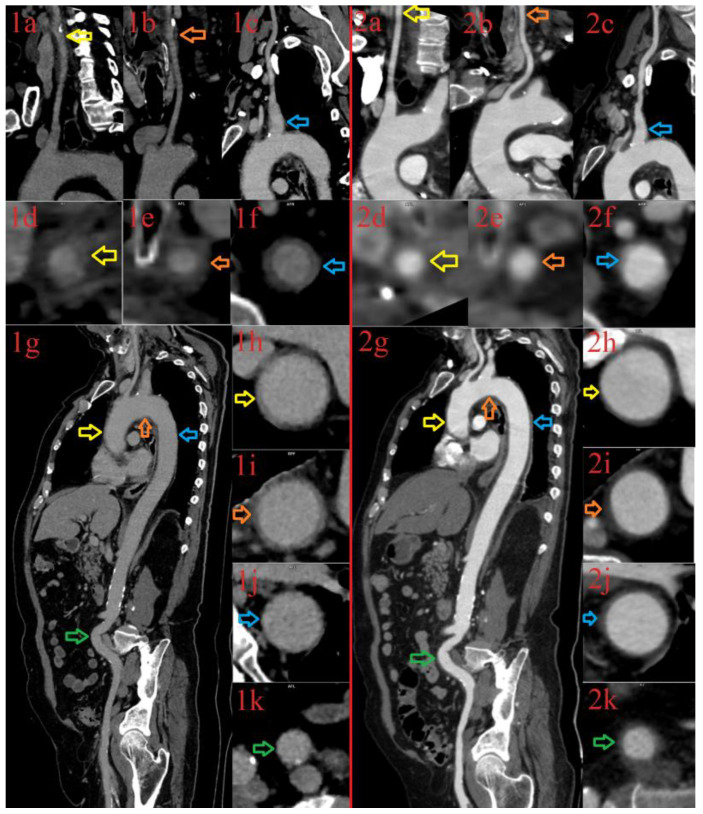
CT aortography of a 69-year-old male with large-vessel vasculitis performed at baseline (Images (1a–1k)) and at the six months follow-up examination (Images (2a–2k)). The baseline CT depicts wall thickening of the aortic arch branches that is barely seen after six months in the right carotid artery (Baseline: Image (1a,1d); Follow-up: Image (2a,2d); yellow arrows), the left carotid artery (Baseline: Image (1b,1e), Follow-up: Image (2b,2e); orange arrows), and the left subclavian artery (Baseline:: Image (1c,1f); Follow-up: Image (2c,2f); blue arrows). In addition, the baseline CT aortography depicts the inflammation of the ascending aorta (Image (1g,1h), yellow arrows) and the aortic arch (Image (1g,1i), orange arrows), which was significantly thinner after six months (The ascending aorta: Image (2g,2h), yellow arrows; The aortic arch: Image (2g,2i), orange arrows). On the contrary, the descending thoracic aorta (Image (1g,1j), blue arrows) and iliac arteries (Image (1g,1k), green arrows) are intact at baseline CT, and appeared inflamed after six months (The descending thoracic aorta: Image (2g,2j), blue arrows; Iliac arteries: Image (2g,2k), green arrows).
